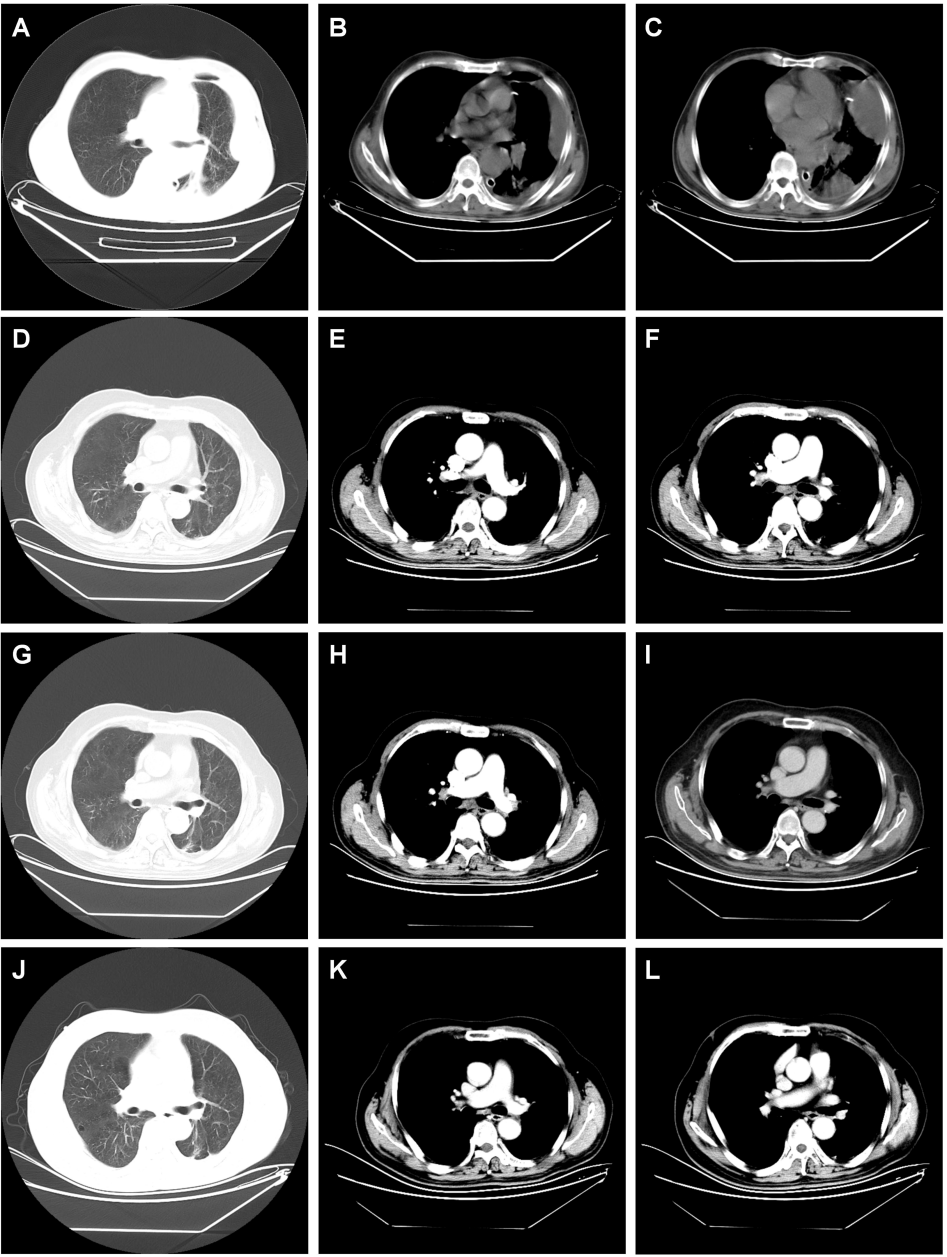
Dynamic imaging of pulmonary and pleural lesions across different stages. (A–C) The postoperative chest CT scan performed on May 3, 2021 showed the giant mass in the left lower lobe of the lung (diameter 32 mm); left pleura, left chest wall have several metastases; (D–F) chest CT scan performed on November 13, 2021 after the 6th cycle of combined therapy showed complete resolution of pulmonary and pleural lesions. (G–I) Chest CT scan on September 14, 2023 after completing two years of monotherapy with tislelizumab showed sustained complete remission with no signs of recurrence. (J–L) Chest CT scan on March 25, 2025 showed sustained complete remission with no signs of recurrence, indicating a progression-free survival (PFS) of 46.5 months. note: the red arrow marks the location of the lesion.
